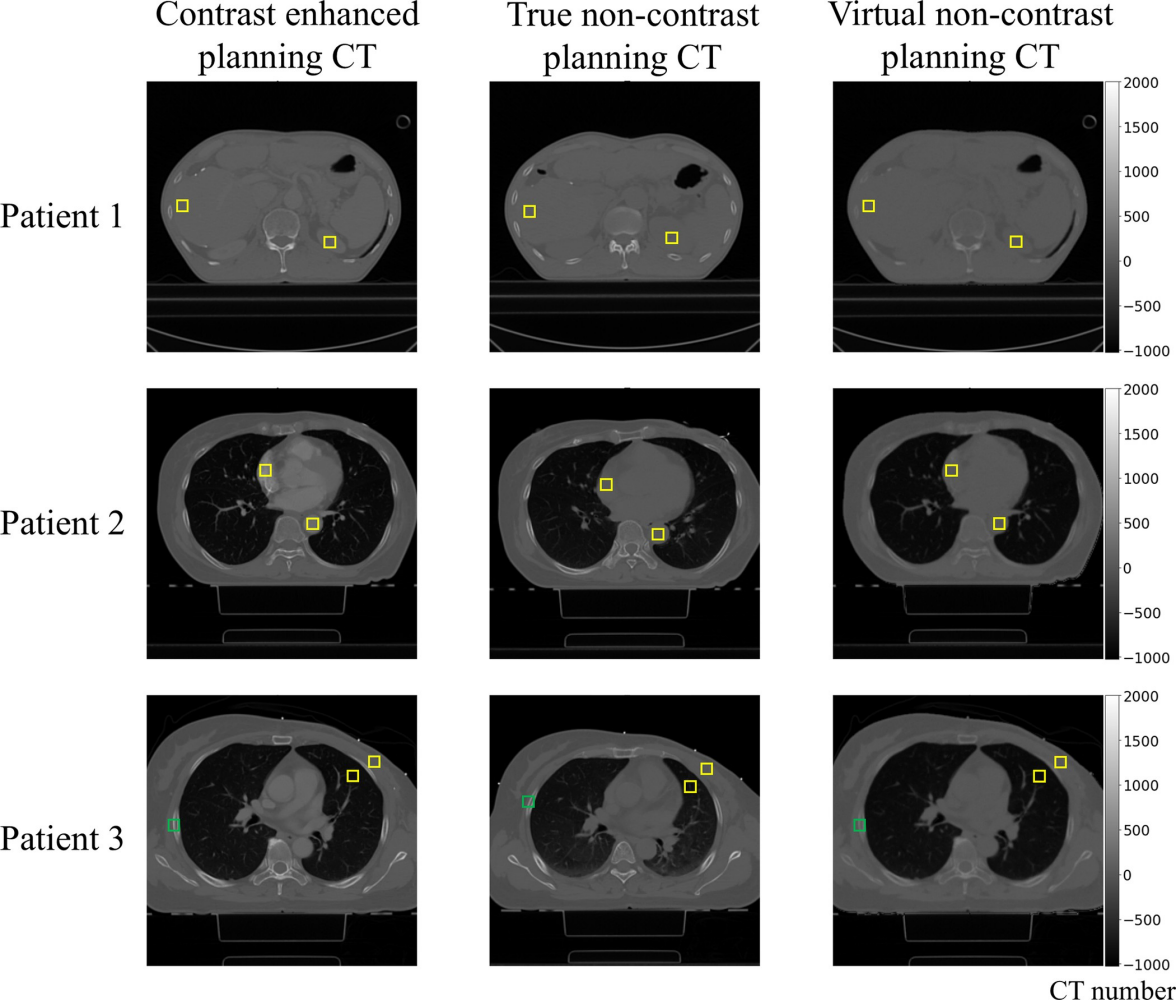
ROI analysis of the VNC-pCT results. In the top row, the ROI on the left side of each image is the liver, and the ROI on the right side is the kidney. In the middle row, the ROI in the upper left side of each image is the right atrium, and the ROI in the lower right side is the descending aorta. Lastly, in the bottom row, the ROI marked with a green box represents the ribs, while among the two ROIs marked with yellow boxes, the one on the left is the lung and the one on the right is the breast. ROI, region of interest; VNC-pCT, virtual non-contrast planning computed tomography.
